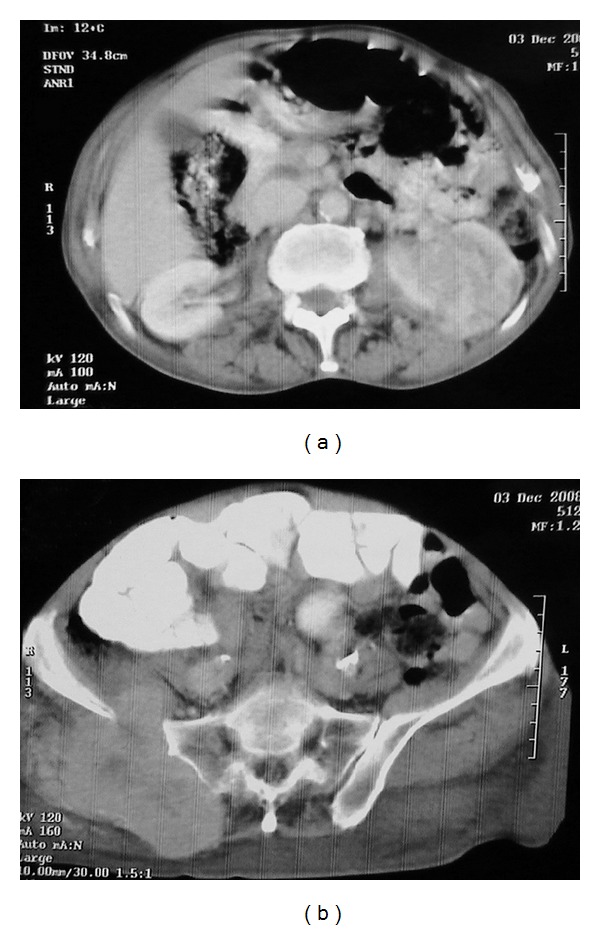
Abdominopelvic CT: (a) image showing 8 cm large mass on the left kidney and (b) CT image demonstrating 11 × 11 × 8.5 cm giant gluteal mass causing the destruction of the iliac bone and acetabulum.
